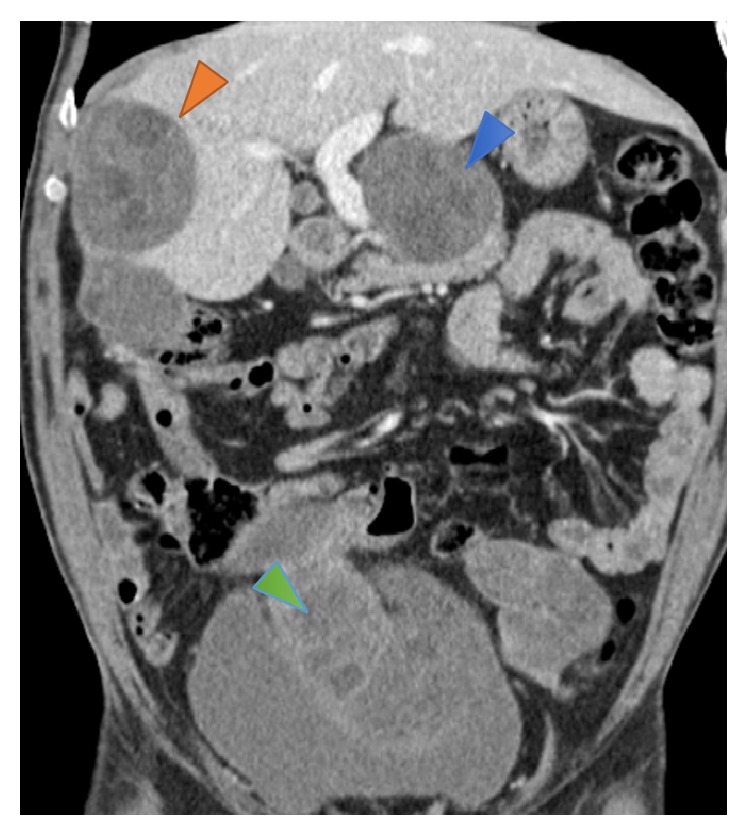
Coronal contrast enhanced CT image shows multiple abdominal hypodense lesions within liver (orange arrowhead) and lesser omentum (blue arrowhead) and peritoneum and above the bladder (green arrowhead).
